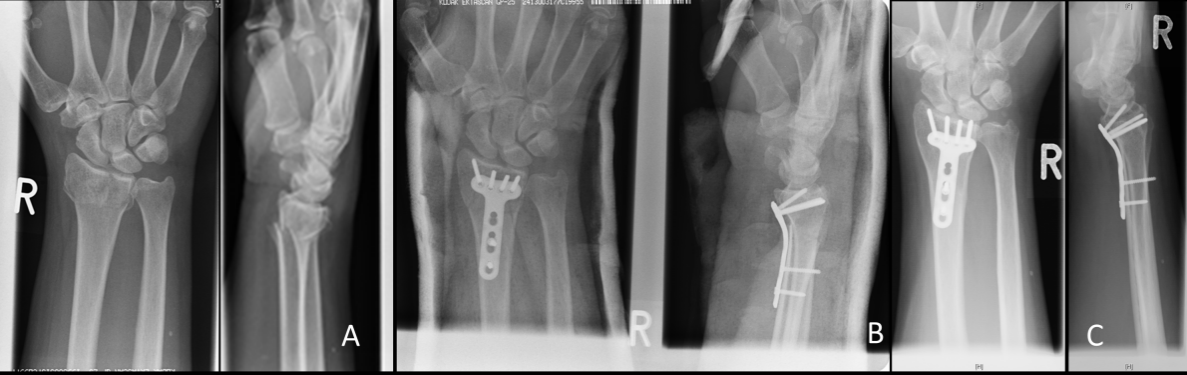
Radiographic follow-up series of a 52-year-old female patient with an AO 23C2 fracture, treated through a volar approach with 2.4 mm LCP (Synthes^®^). A) Posttraumatic x-ray. B) Follow-up radiographs after 12 weeks. C) Follow-up radiograph 16 months after trauma with no major loss of reduction.
